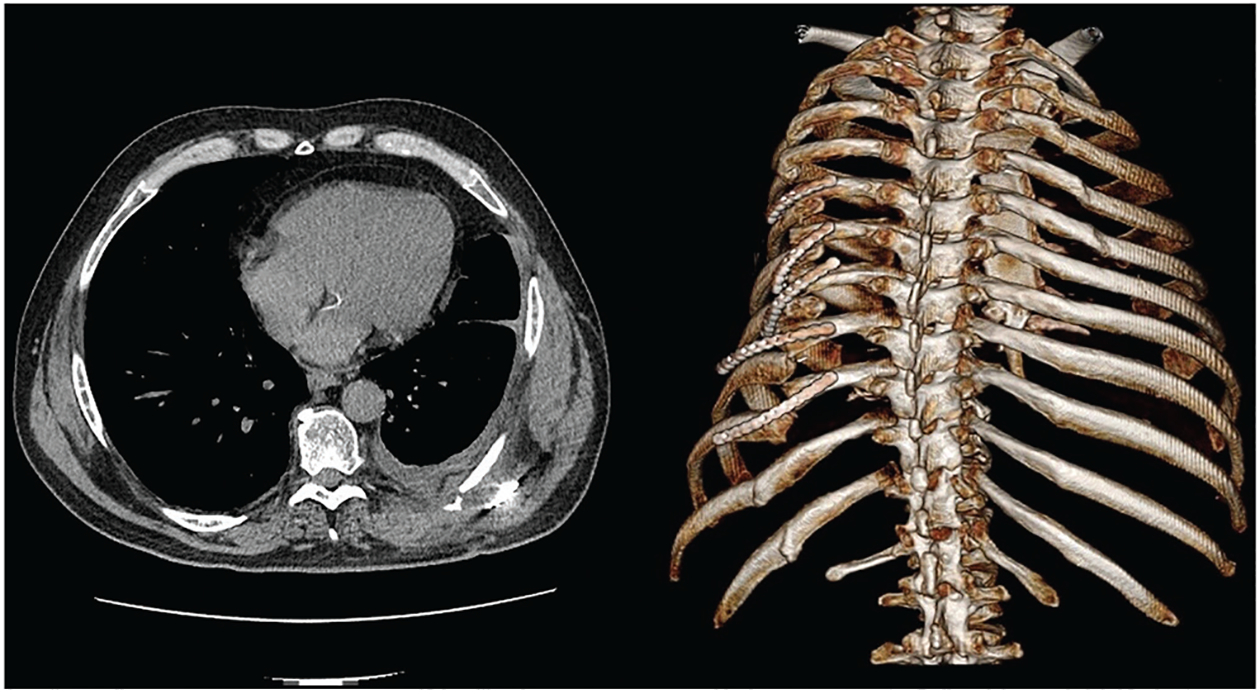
Computed tomography demonstrating hardware failure with pull out of the screws from rib stump and chest wall collapse
